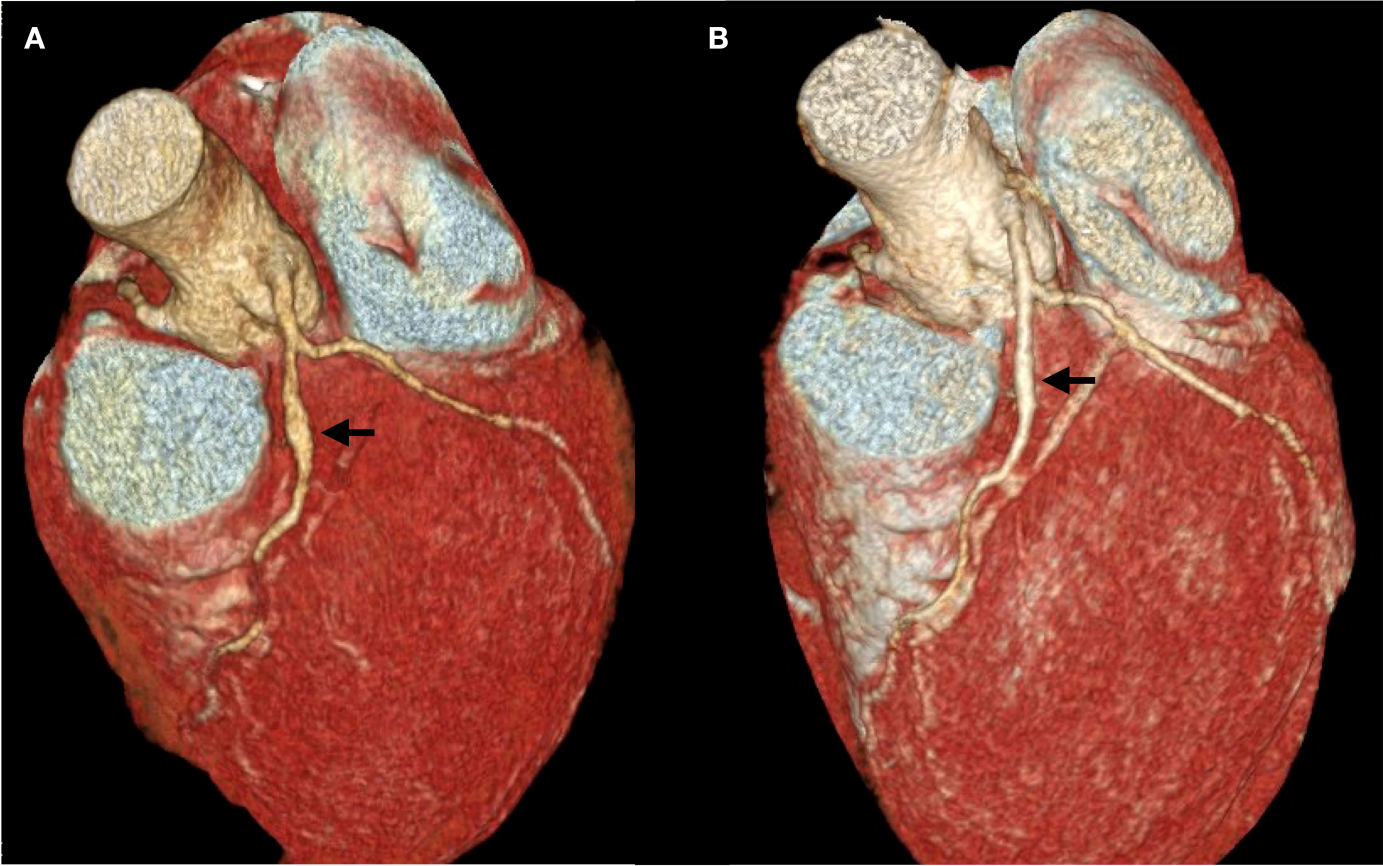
Computed Tomography Coronary Angiography. (A) The first exam was performed on April 27, 13 days after the onset of the disease, 6 days after tocilizumab administration and 3 days after intravenous immunoglobulins. A 5 mm fusiform ectasia is present at the proximal part of the anterior interventricular coronary artery (arrow). The external diameter of the artery is 3 mm above and below the ectasia; dilation area 19,3 mm^2^. (B) Second exam, performed on May 14, demonstrating a marked decrease in aneurism size. Four millimeter fusiform ectasia; dilation area 10,6 mm^2^.
